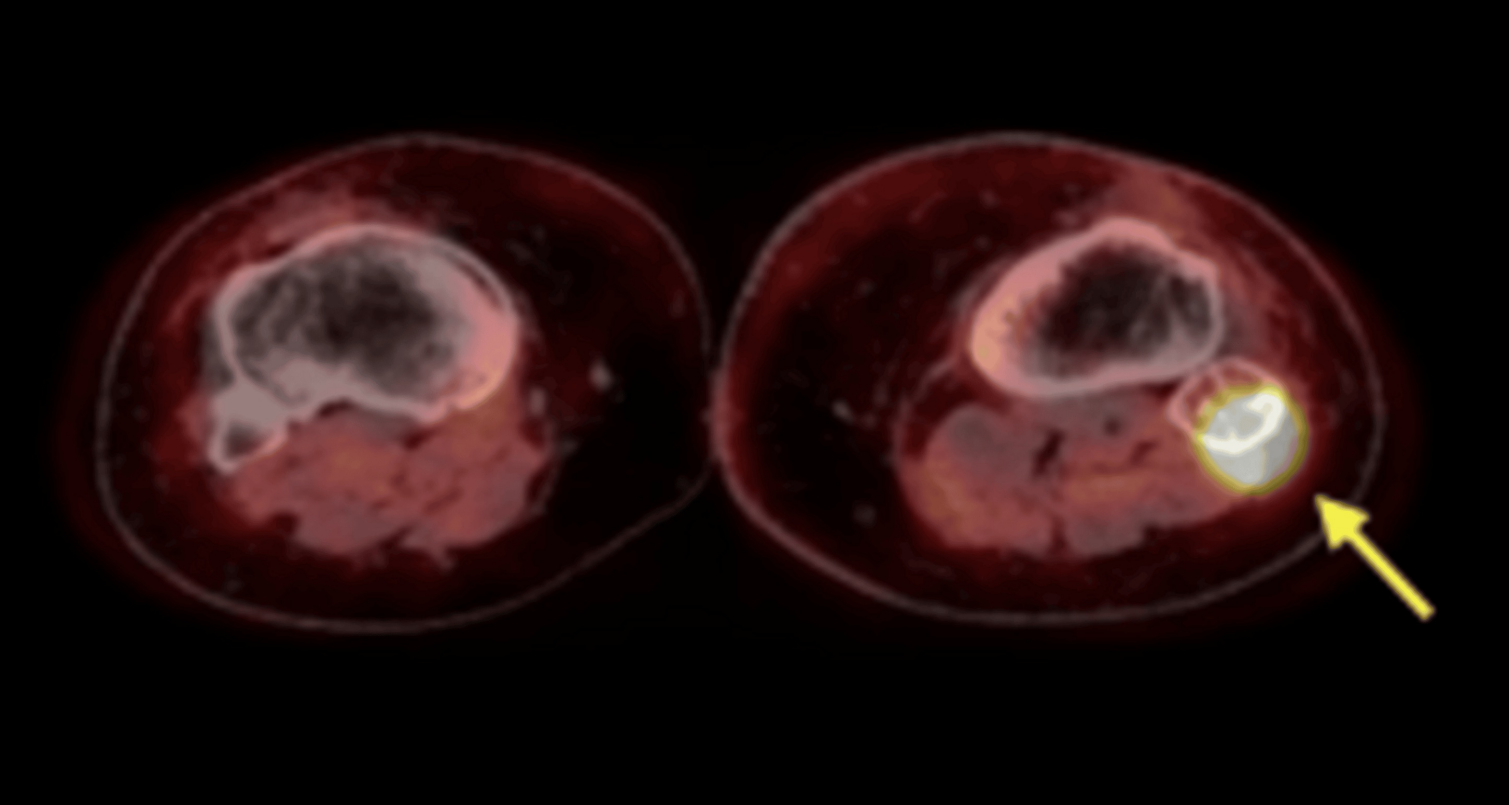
PET-CT scan (axial view): FDG-avid lytic lesion in the head of the left fibula (arrow)
